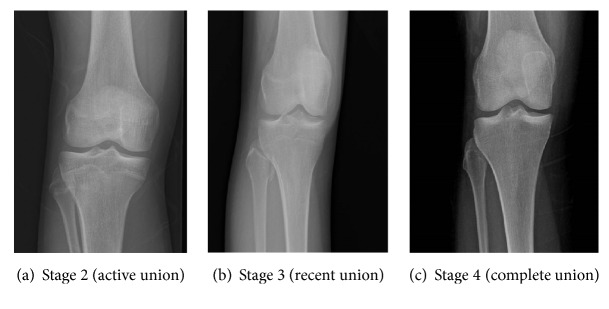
Radiographic images of knee joint showing stage 2 labeled as (a) and stage 3 labeled as (b) and stage 4 labeled as (c).
